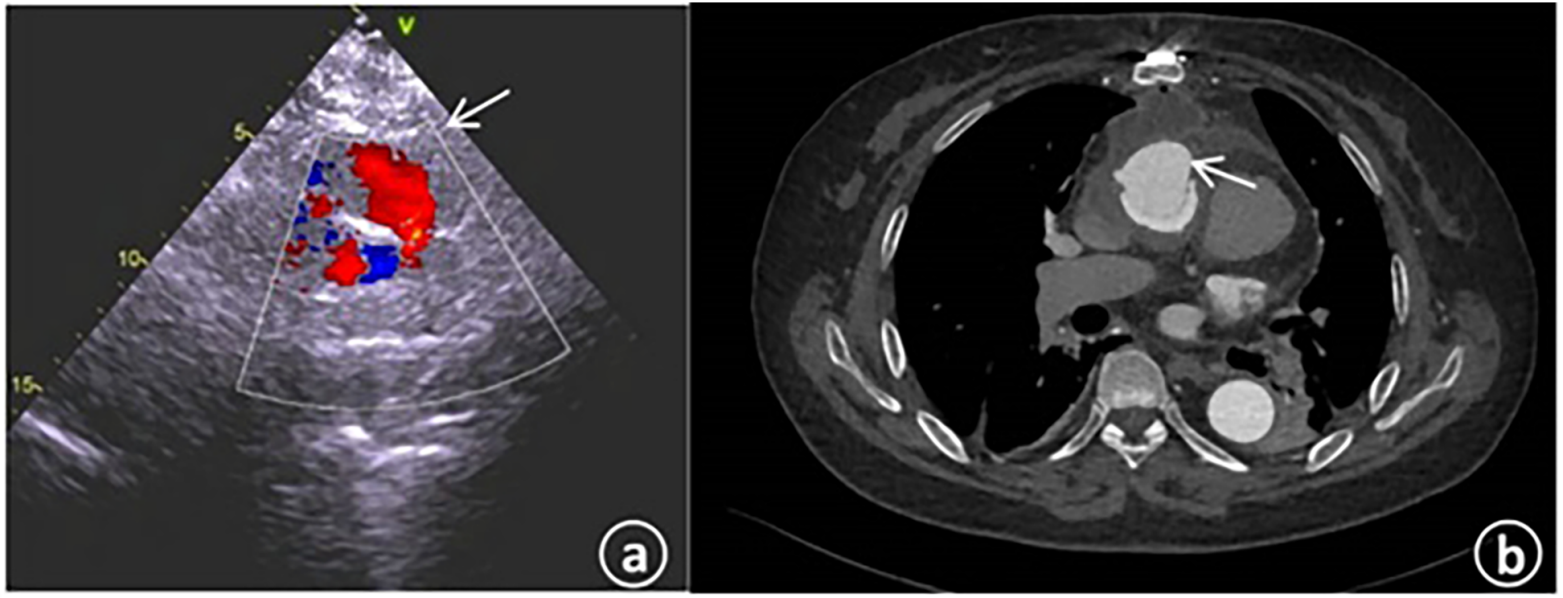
(a) The cardiac color – Doppler ultrasound upon admission revealed the presence of abnormal blood flow within the artificial blood vessel located on the posterior wall of the distal ascending aorta. (b) The computed tomography angiography of the aorta during admission indicated the existence of patchy contrast agent shadows beside the ascending aorta, with local communication to the right atrial appendage.
